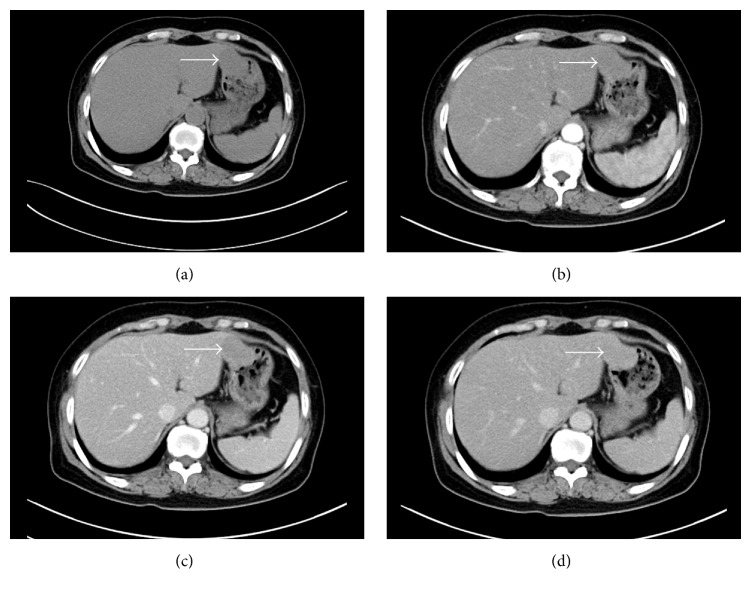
Computed tomography (CT) image of the gastric schwannoma. (a) An oval-shaped mass (white arrow) with a size of 43 × 32 mm was observed in the lesser curvature of the stomach, which exhibited a slightly low density on plain scanning CT imaging. (b) The CT value of the mass was about 38 HU before the injection of contrast medium. The mass showed delayed enhancement with a CT value of 53 HU on arterial-phase enhanced CT scanning. (c) The CT value of the mass was slightly increased (68 HU) on portal venous-phase enhanced CT imaging. (d) The CT value of the mass was increased (78 HU) on delayed enhanced CT scanning after a delay of 3 minutes.
